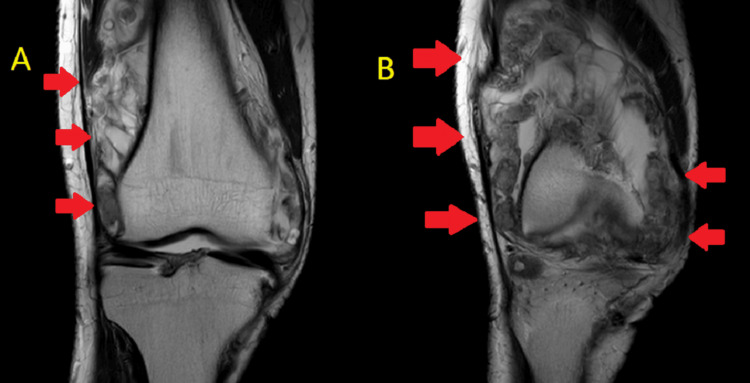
Coronal MRI sections (A) and (B) showing multiple loose bodies T2-weighted images (A) and (B) show multiple loose bodies circumferentially around the knee MRI: magnetic resonance imaging
